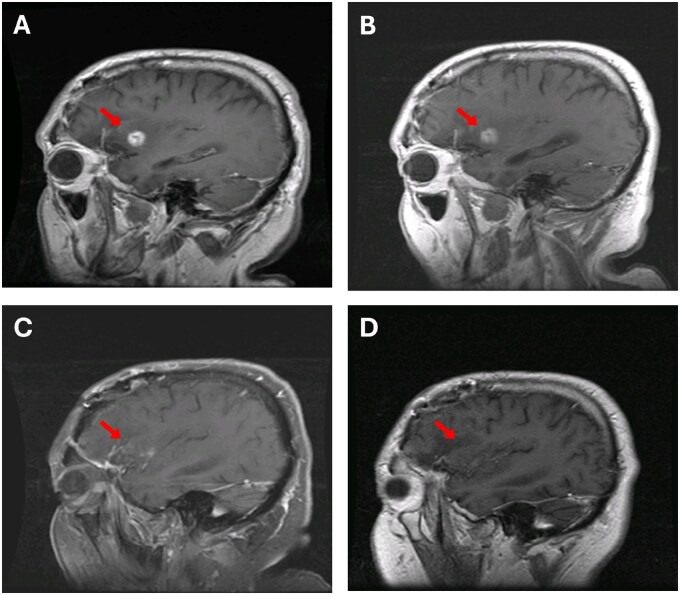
MRI of patient achieving complete response after 7 cycles of berubicin. A 52-year-old man with recurrent GBM that had progressed from AA experienced a complete response after 7 cycles of berubicin at a dose of 2.4 mg/m^2^/day. This CR was confirmed via follow-up MRI. (A) Before berubicin (January 20, 2006). (B) After 2 cycles (March 7, 2006). (C) After 6 cycles (July 11, 2006). (D) After 7 cycles (August 1, 2006). AA, anaplastic astrocytoma; CR, complete response; GBM, glioblastoma multiforme; MRI, magnetic resonance imaging.
